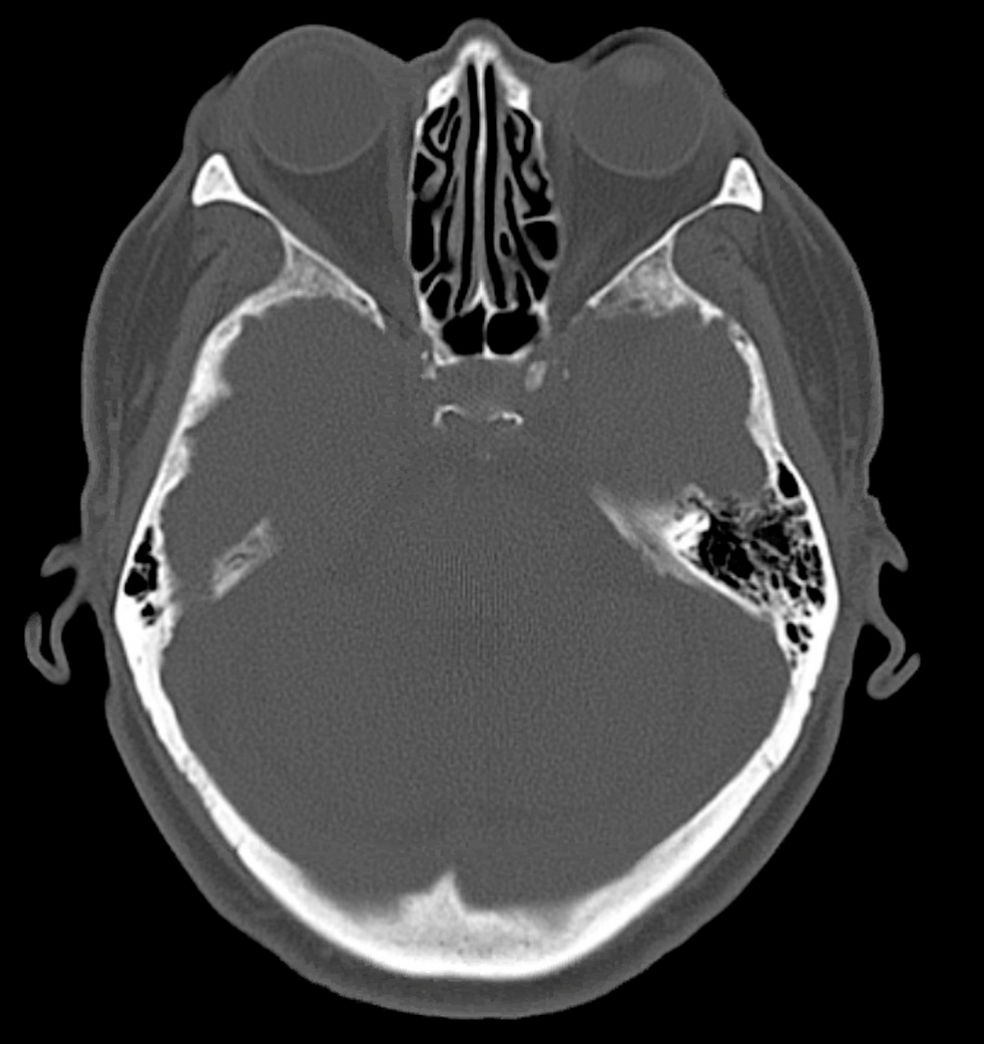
Selected CT axial image of the head without contrast demonstrating sparing of lateral recti and medial recti muscle enlargement CT: computed tomography
